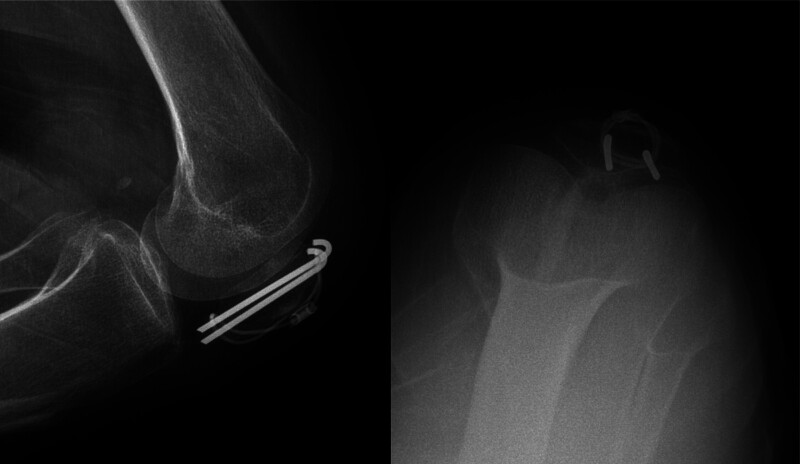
X-ray results showed that the fracture had healed very well without hardware failure at 7th month postoperatively.
